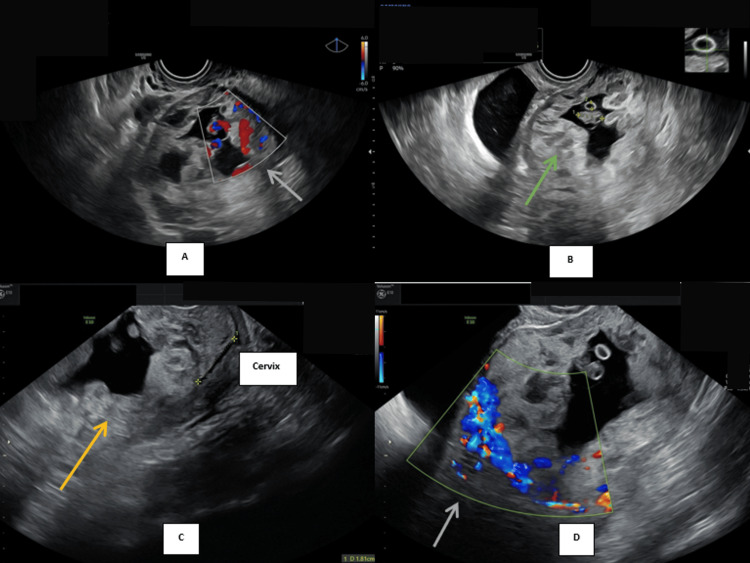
(A&D) Transvaginal ultrasound Doppler image (grey arrows) showing vascularized vesicles within the gestation sac. (B) Sac containing vesicles (green arrow) seen with fetal echo at the lower pole of the uterus. (C) Location of the sac (yellow arrow) in relation to the cervix
